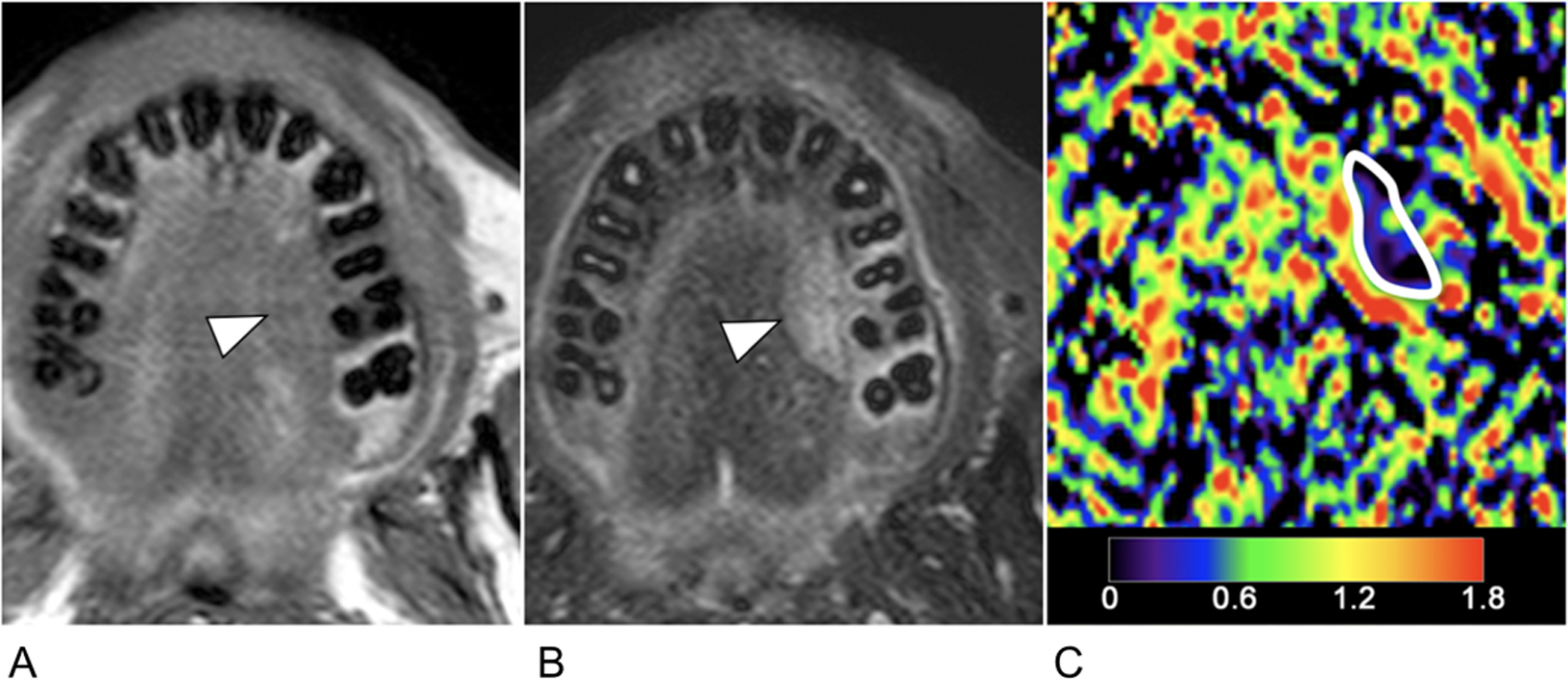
39-year-old man with small cell carcinoma (T3N1M0). A, Axial T1-weighted MR image shows ill-defined, homogeneous cancer arising in palatal gland (arrowhead). B, Axial fat-suppressed T2-weighted MR image shows heterogeneous parenchyma (arrowhead). C, Axial, color ADC map. White demarcation indicates an ROI manually placed within the tumor area for ADC measurement. Color scale bar indicates ADC levels (0–1.8 × 10^−3^ mm^2^/s). He died of cancer within the first 3 years (14 months) after surgical excision of primary cancer.
